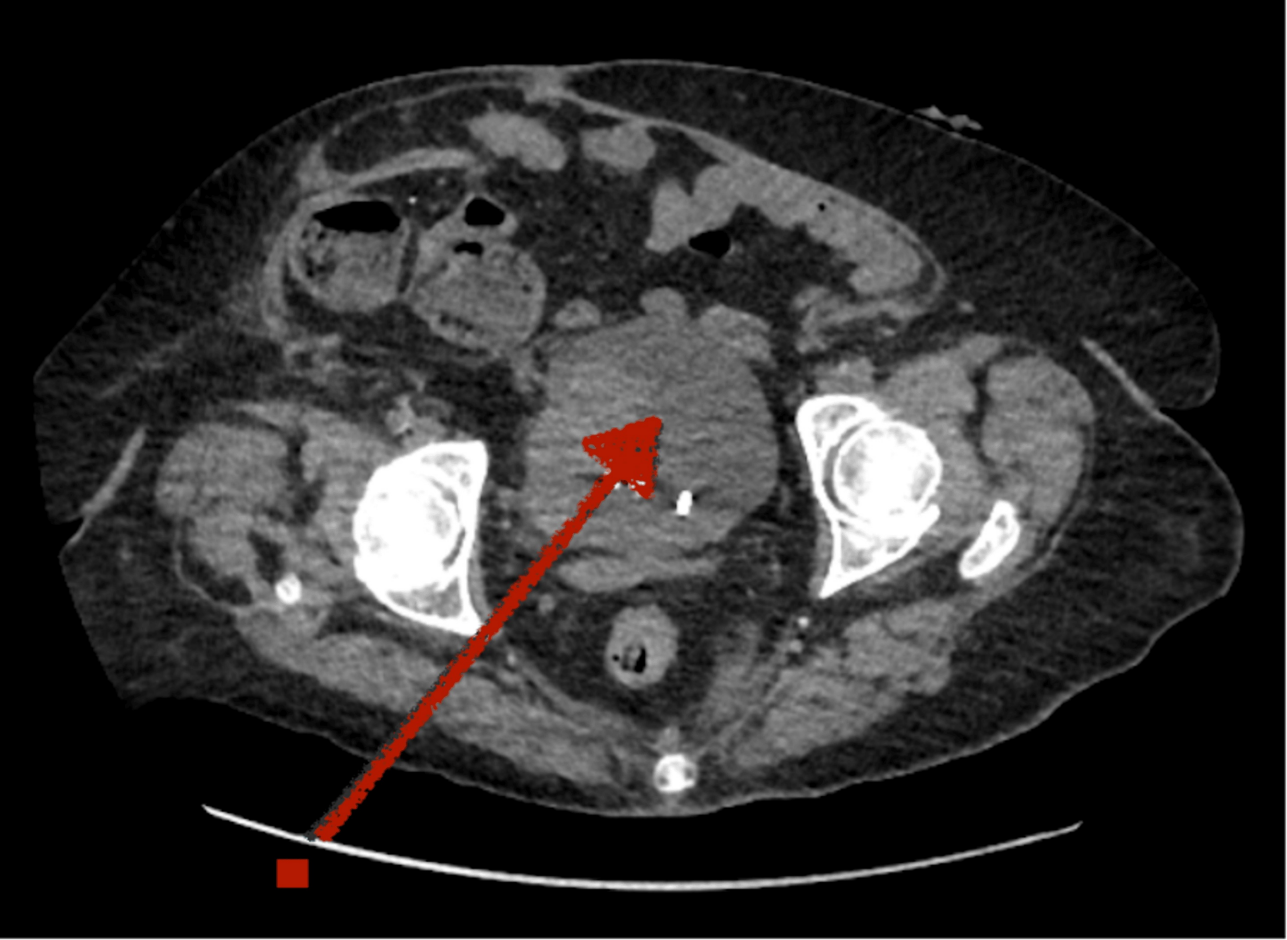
CT urinary tract, showing suspected bladder invasion (red arrow) CT: computed tomography
